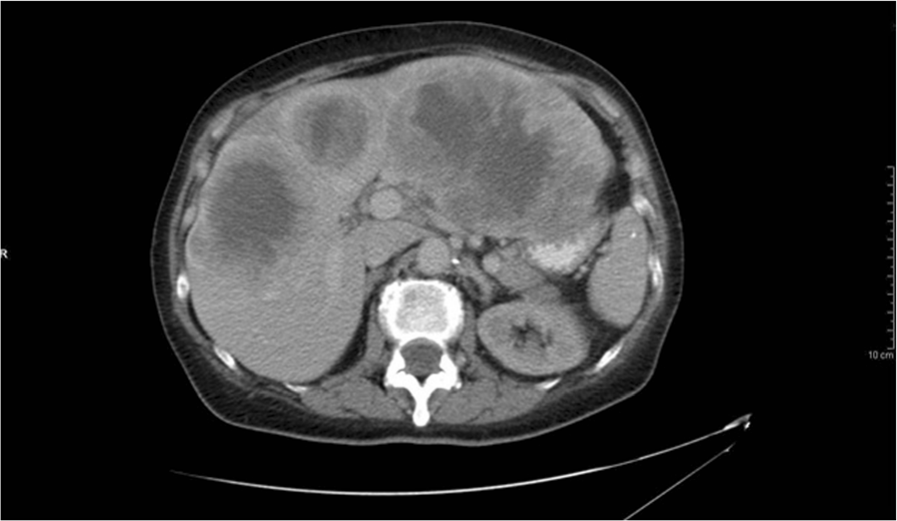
Computed tomography scan of the abdomen demonstrating metastatic solitary fibrous tumor involvement of the liver.
